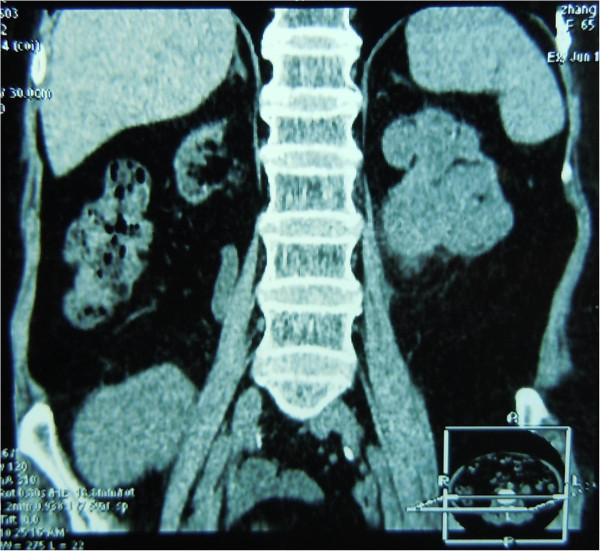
Computed tomography (CT) scan revealed a 5 cm × 4 cm lesion in the left pelvic region.
